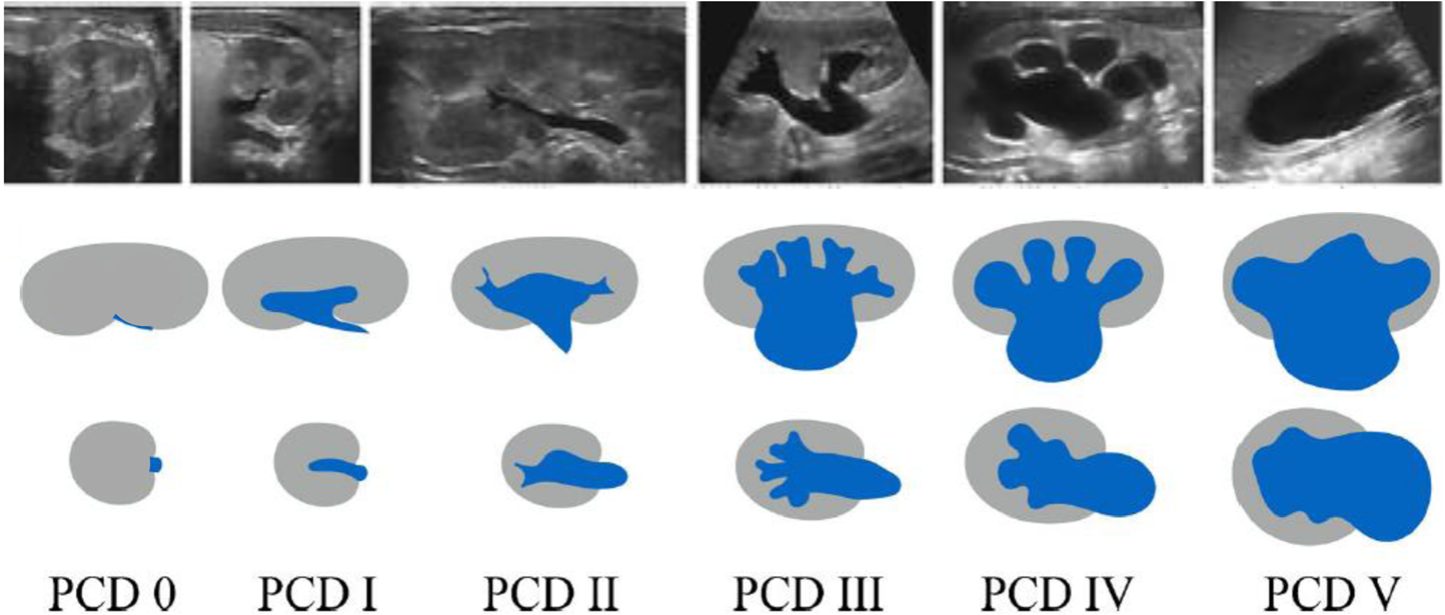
Sonographic grading of postnatal pelvicalycael distention/dilatation (PCD), formerly called the hydronephrosis grading system. *Top row*: longitudinal section. *Bottom row*: transverse section at the level of the renal pelvis. *PCR* 0 collecting system *PCD 0* collecting system not, or hardly, visible — normal; *PCD I* only renal pelvis clearly visible, calices not depictable, axial pelvic diameter<7 mm — considered normal; *PCD II* axial renal pelvis diameter<10 mm, (some) calices visible but with normal forniceal and papillary shape/configuration; *PCD III* marked dilatation of calices and pelvis, pelvic axial width usually >10 mm with flattened papilla and rounded fornices but without parenchymal thinning; *PCD IV* gross dilatation of entire collecting system and thinning of renal parenchyma; *PCD V* used in some places to communicate an extreme PCD IV with only thin, membrane-like residual renal parenchymal rim
